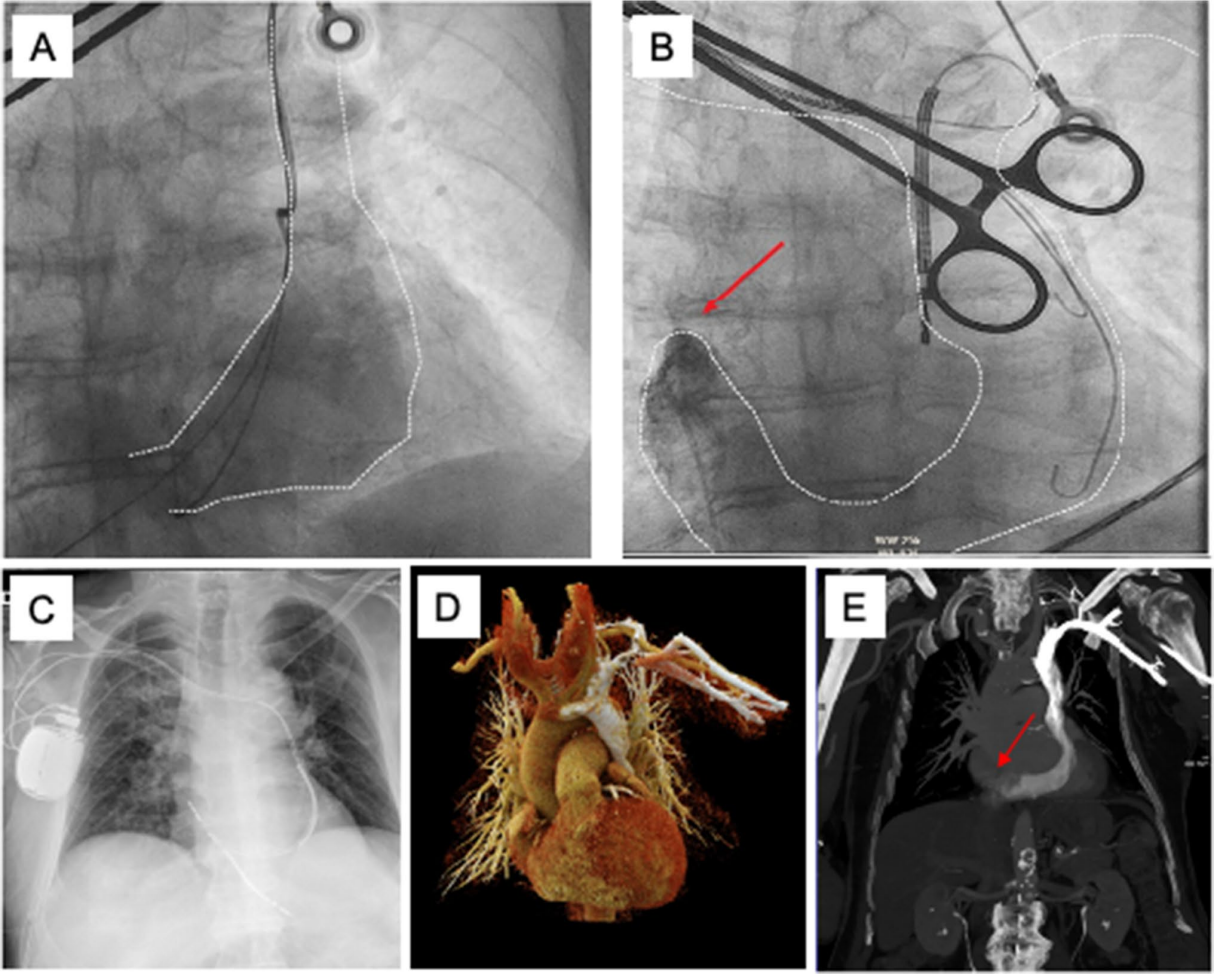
Case # 6: initial non-selective angiography showing a largely dilated CS outlined by the dotted lines, postero-anterior (A). Venous angiography via femoral access confirming the presence of an isolated PLSVC and showing the dilated CS (dotted lines and red arrow). Guidewires and RV-lead were inserted from the right side and are also visible, postero-anterior (B). Chest X-ray after dual chamber ICD implantation (C). 3D reconstruction of chest CT-scan showing the venous anomaly (PLSVC type II) (D). Conventional chest CT-scan with the missing right-sided superior vena cava (red arrow) (E)
